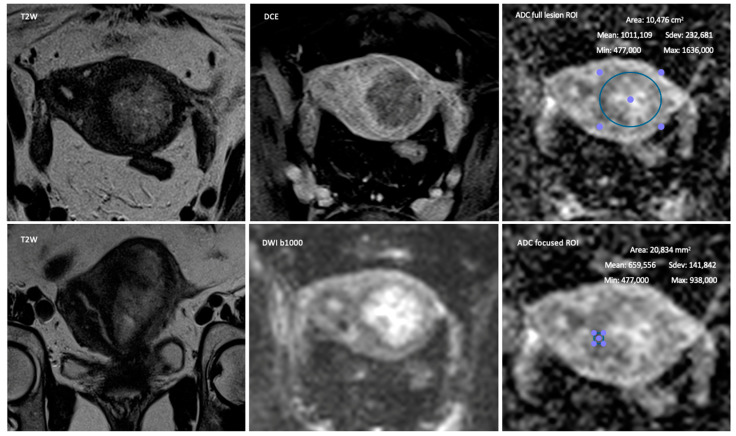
Fifty-seven-year-old female with a Mullerian malformation (septate uterus) staged at MRI as T1b and proven at pathology as T1b. The presence of a uterine malformation is a pitfall for the detection of myometrial infiltration by the tumor. Depiction of the method used for the quantitative data collection with the two different ROI.
